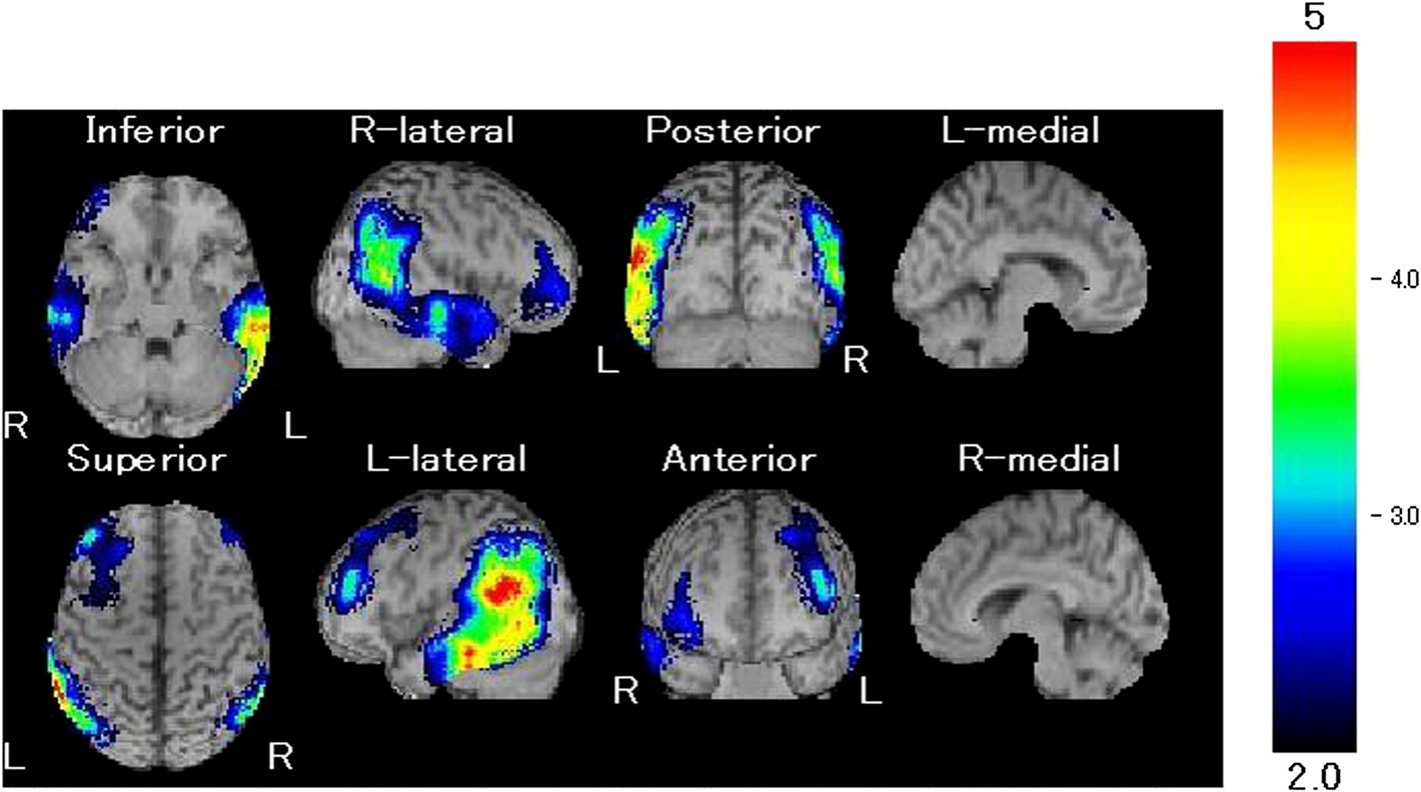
Brain SPECT eZIS analysis of case 1. This series of scans showed relative hypoperfusion mainly in the bilateral temporo-parietal areas.
